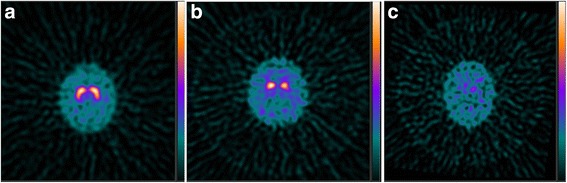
DaT scan examples. DaT scan transaxial SPECT sections for: (a) a patient with essential tremor, showing high symmetric uptake in both caudate and putamen regions; (b) a patient with Parkinson’s disease, showing markedly reduced putamen activity and asymmetric caudate activity; (c) a patient with dementia with Lewy bodies, showing nearly absent caudate and putamen uptake
